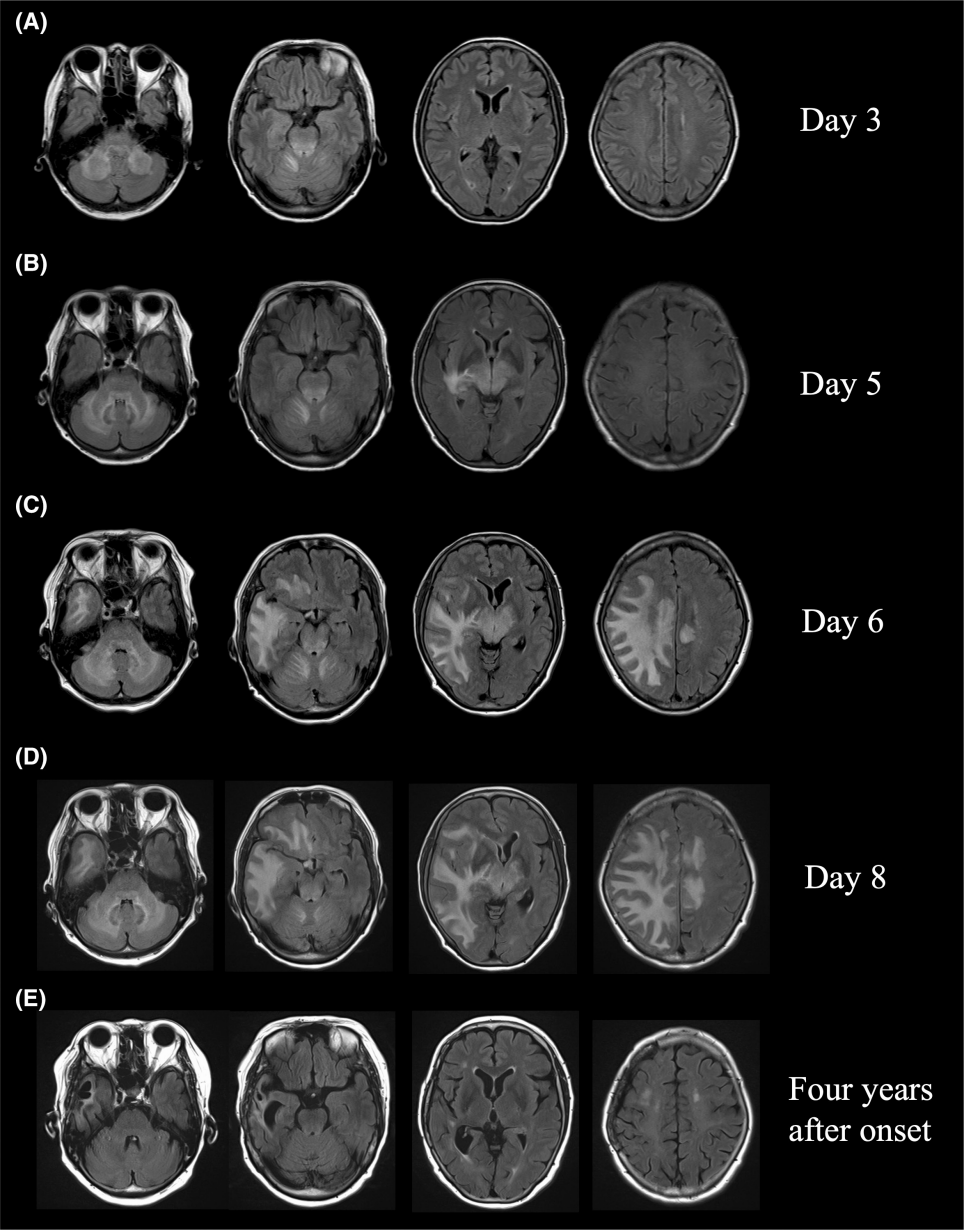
Brain MRI. Fluid attenuated inversion recovery (FLAIR) images show T2 high‐signal intensity lesions around the dentate nuclei, cerebellar peduncles, the right ventral side of the medulla oblongata, and tegmentum (Day 3, A). A new lesion is detected in the right temporal lobe (Day 5, B). The lesions expand rapidly, and extensive T2 high‐signal intensity lesions are observed in the bilateral cerebellar hemispheres, brainstem, bilateral thalami, right basal ganglia, right posterior limb of the internal capsule, and subcortical cerebral hemispheres. The abnormal signal area also extends to the left cingulate gyrus via the body of the corpus callosum. The lesion has a mass effect; the right ventricle is slightly deformed, and the median structure is deviated to the left (Day 6, C). The lesion has enlarged further, and the brain swelling is aggravated (Day 8, D). The brain swelling has improved, and the lesion has shrunk (4 years after onset, E).
